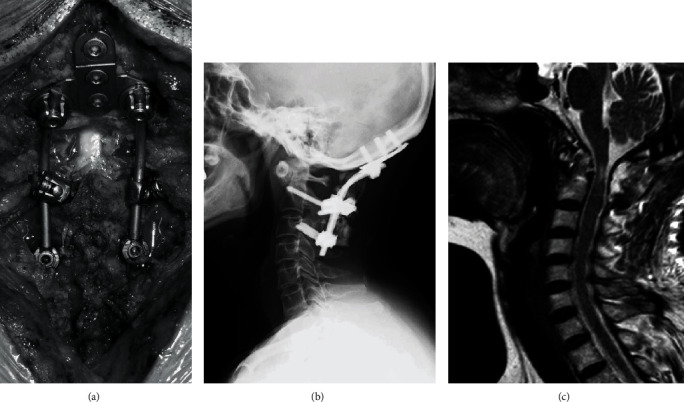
Postoperative image. Posterior decompression fusion from the occipital bone to C3 was performed (a). Side view X-rays show successful placement of the implant as indicated (b). MRI reveals that the compression of the spinal cord has been released by C1 laminectomy (c).
